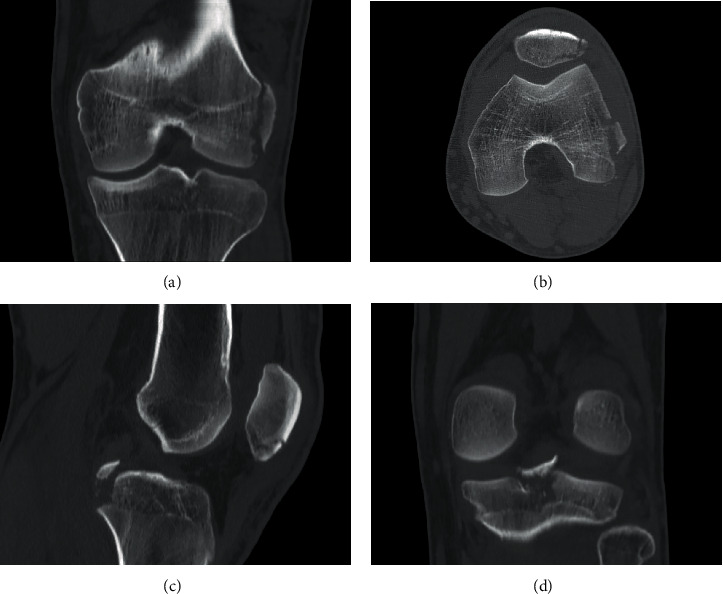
Coronal (a, d), axial (b), and sagittal (c) CT scan on admission to the Emergency Department.
